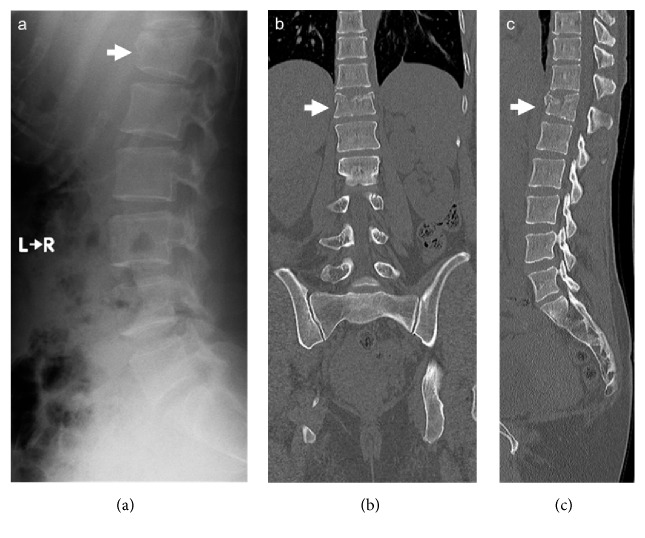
(a) Roentgenogram (a) and CT (b and c) showing compression fracture of Th12 (white arrows).
